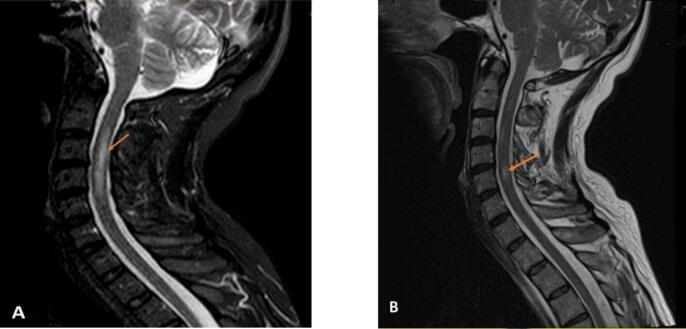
Neuroimage of the patient. A and B: T2-weighted magnetic resonance imaging (MRI). These images show hyperintense signal lesion within the C2-C5 cervical spinal cord and C5 cervical spinal cord.
